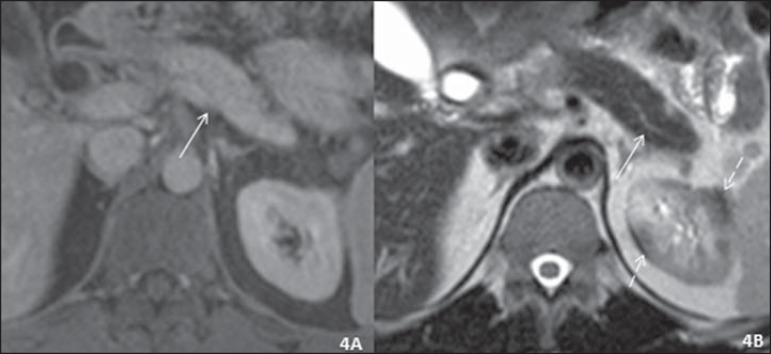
Axial abdominal MRI scan taken after corticosteroid therapy.
